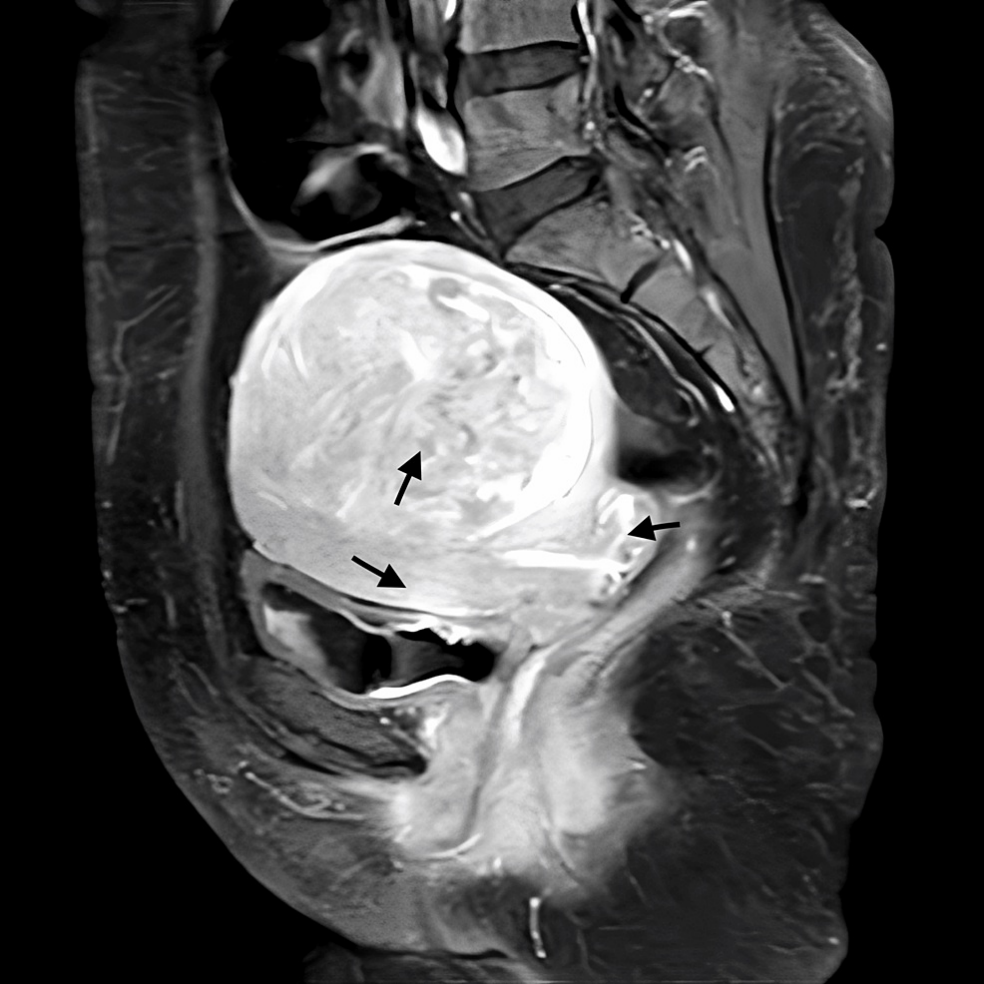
Case 3- MRI-pelvis (plain and contrast) T1 fat-saturated (T1FS) post-contrast showing a hyper-intense lesion with a whorled pattern.
